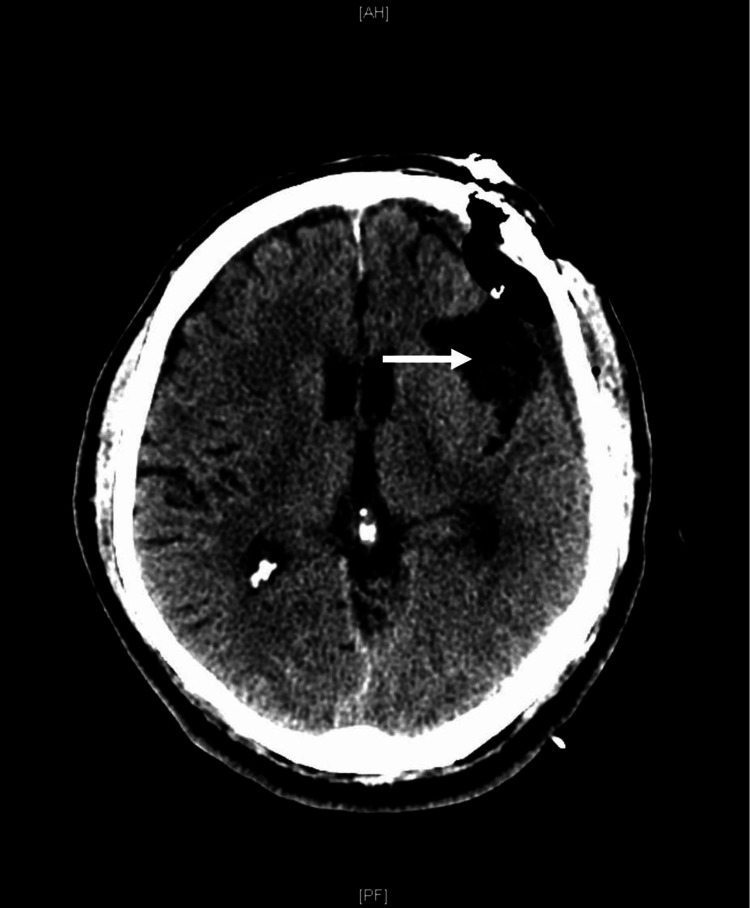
Post-operative CT of head confirms evacuation of subdural hematoma. CT: Computed tomography.
